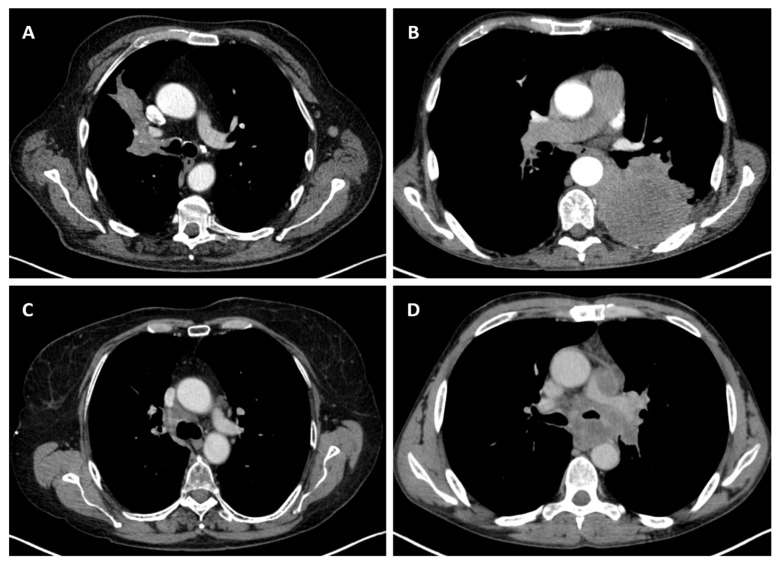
Axial CT of four different cases of small cell lung cancer: (A) right hilar mass with segmental atelectasis and expansion into the right main bronchus; (B) left voluminous mass with central necrosis, pleural invasion and periaortic extension; (C) small-sized central right tumour with paratracheal location; and (D) central left tumour with important mediastinal invasion involving the pulmonary trunk, left pulmonary artery, left main bronchus, and oesophagus.
